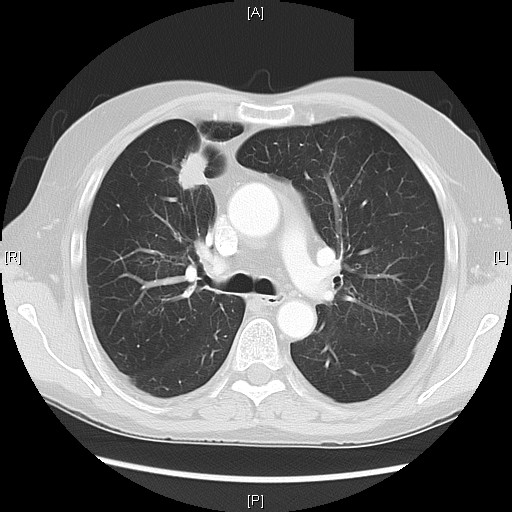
CT scan chest. Lesion in the right lung.
